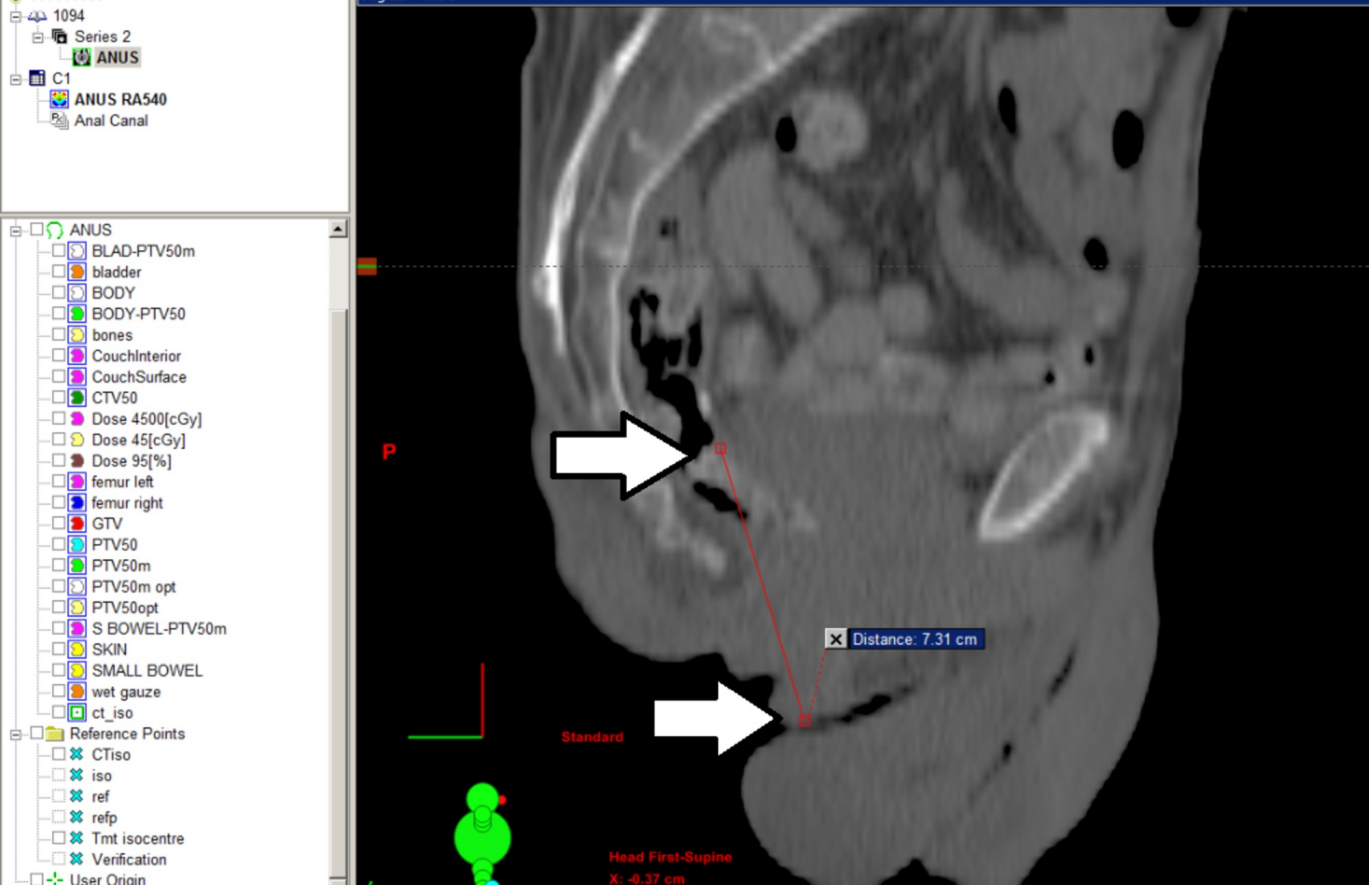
CT imaging showing large tumor over 7 cm in length (white arrows), involving peri-anal skin, the entire anal canal, and lower rectum CT, computed tomography
